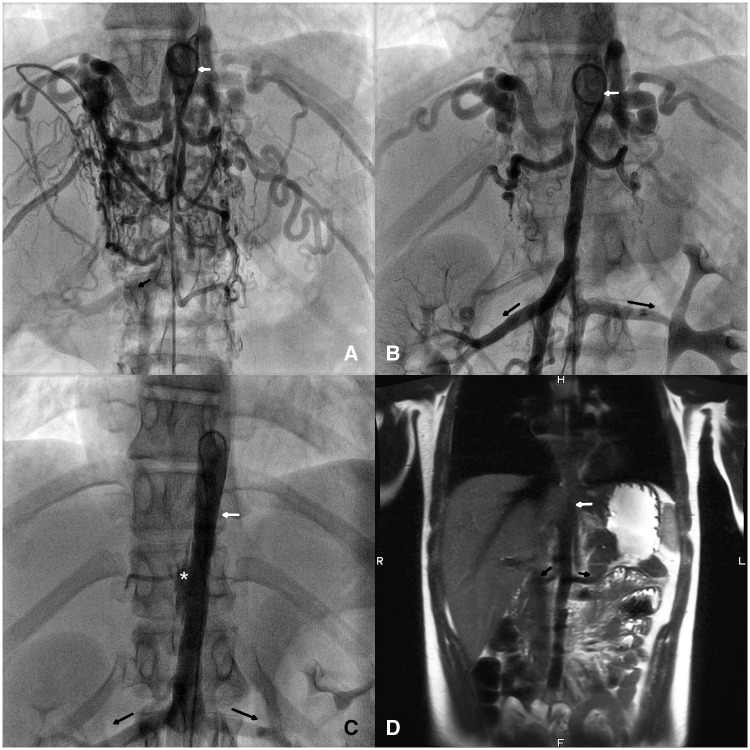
(*A*) Initial presentation of the abdominal aorta with subtotal occlusion caused by a 1.7 mm catheter passing through the stenosis. There is a massive collateralization and only minimal contrast opacification of the right renal artery. The white arrow marks the upper entrance to the tubular coarctation of the abdominal aorta, and the black arrows mark the course of the left and right renal artery, if visible. (*B*) Anatomy after the first dilation with a Paclitaxel covered balloon. (*C*) After the fourth intervention using a 12 mm drug-eluting balloon an endothelial lesion and intra mural contrast was noted (white asterisk). (*D*) Magnetic resonance imaging at latest follow-up 2.7 years after this last intervention shows an unobstructed aorta without collateralization.
